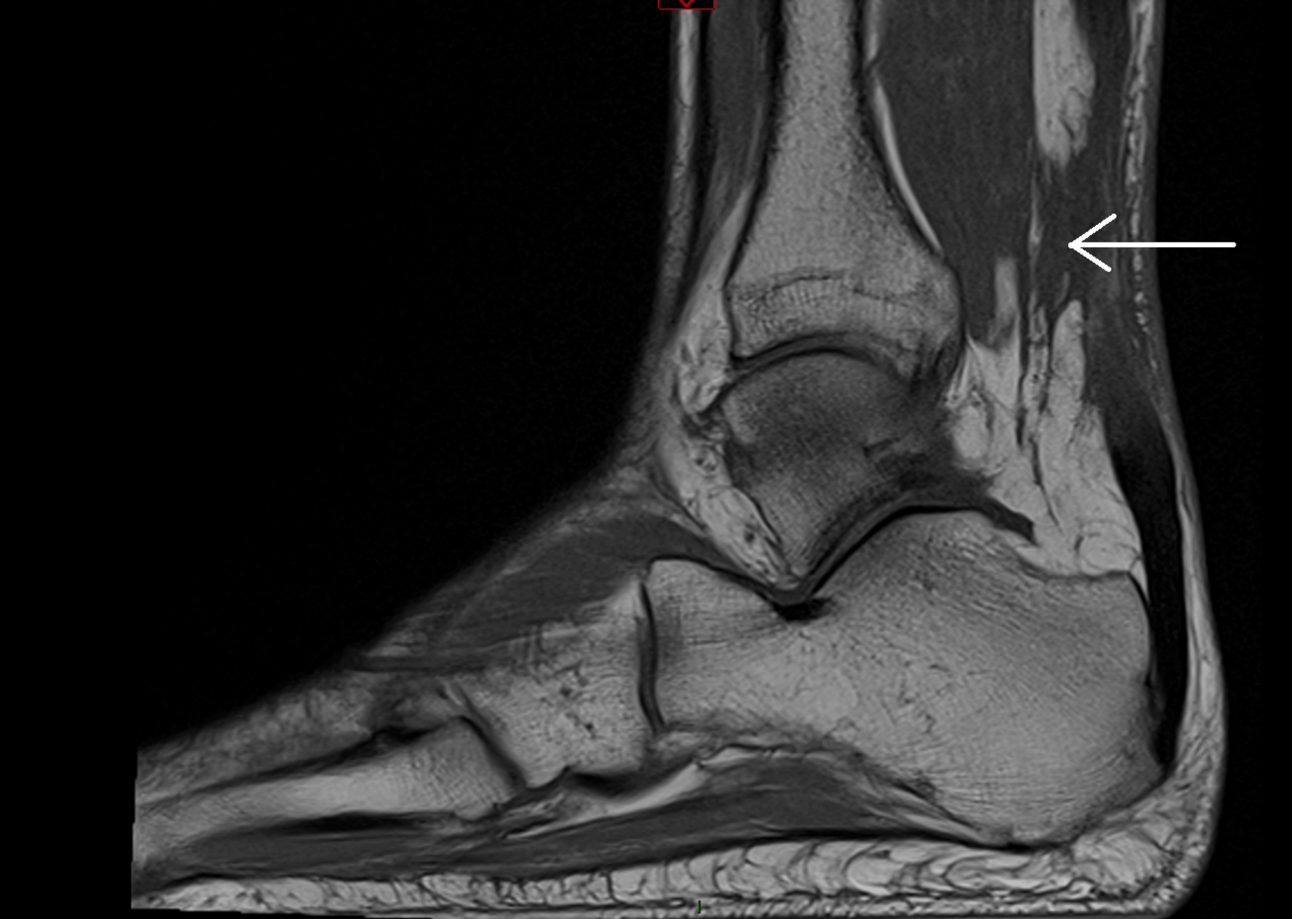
Right ankle MRI – 5 cm gap showing Achilles tendon rupture with local soft tissue inflammatory changes, indicated by white arrow
